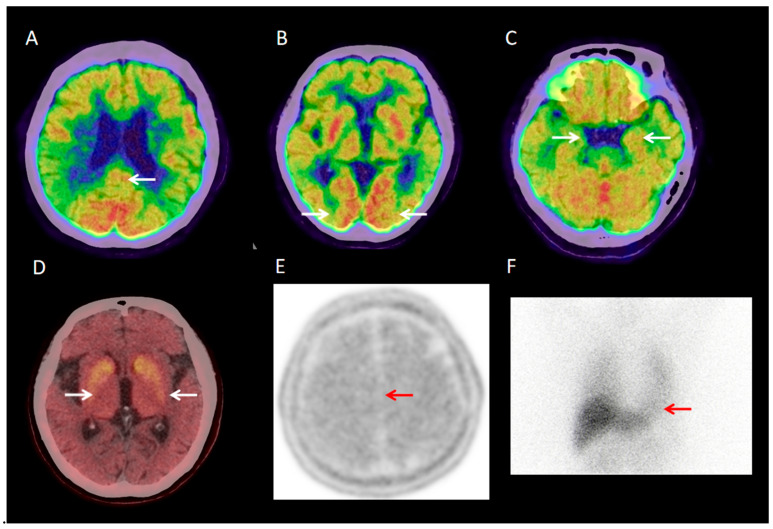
A 71-year-old female patient with 3-year fluctuating cognitive impairment and visual hallucinations. ^18^F-FDG PET/CT shows preserved posterior cingulate metabolism (posterior cingulate island sign), bilateral parieto-occipital hypometabolism, and medial temporal hypometabolism ((A–C); white arrows). ^18^F-FP-CIT PET/CT shows reduced uptake in the bilateral putamen tails ((D); white arrow). ^18^F-AV45 PET shows diffuse cortical Aβ deposition ((E); red arrow). ^131^I-MIBG scintigraphy indicates cardiac sympathetic denervation ((F); red arrow).
